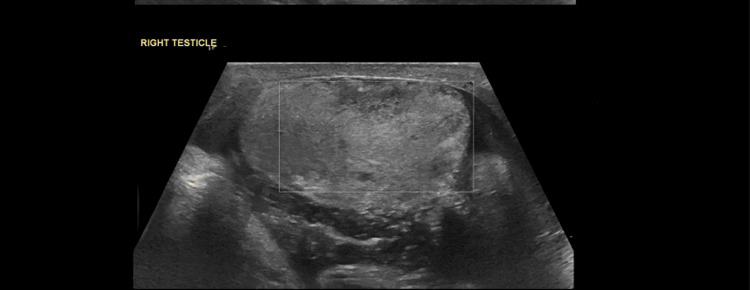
US testis Ultrasound of the right testis showing absent blood supply and early signs of ischemia.
